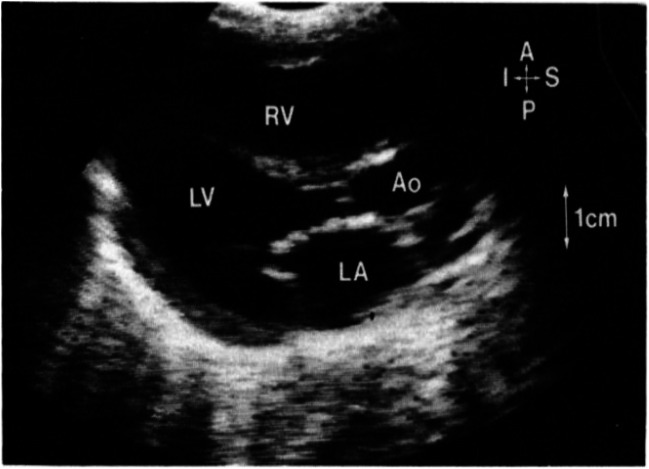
Parasternal long-axis view of neonatal critical aortic stenosis. A hypoplastic aortic valve annulus and post-stenotic dilation of the ascending aorta are noted
